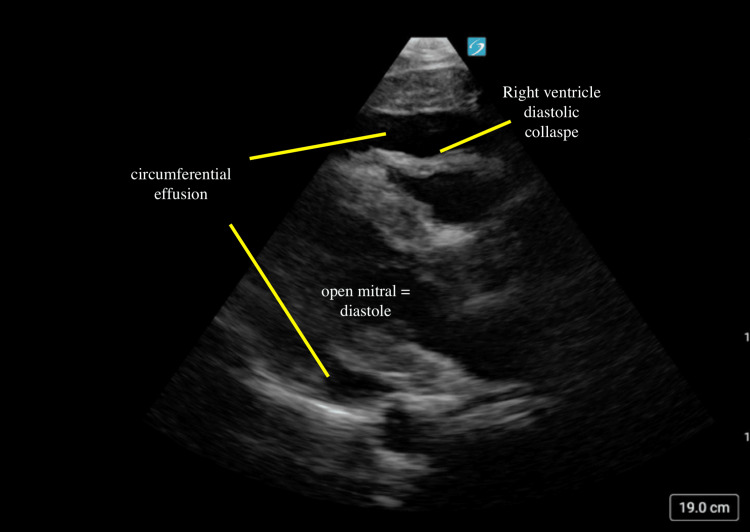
Parasternal long-axis tamponade In this image, a significant circumferential pericardial effusion is seen. Partial collapse of the right ventricle is also seen which occurs during diastole, which is demonstrated by an open mitral valve.
